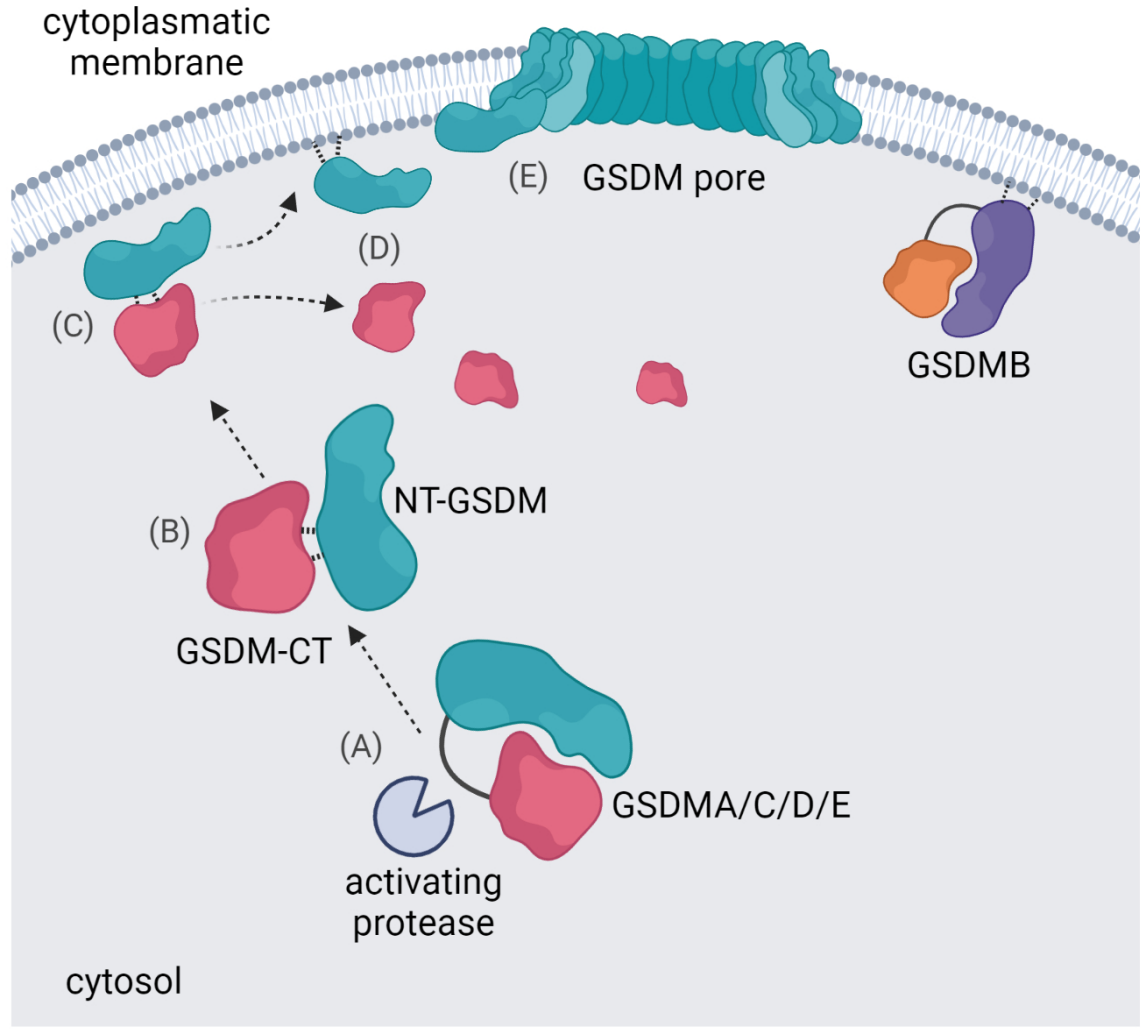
Gasdermin pore formation. An activating protease cleaves the gasdermin protein in the linker region between NT-GSDM and GSDM-CT (A) the NT-GSDM and GSDM-CT subunits remain associated in a complex (B) the NT-GSDM/GSDM-CT complex interacts with a membrane (C) and dissociates upon this interaction (D) NT-GSDM subunits oligomerize and form pores (E) GSDMB has a unique ability to interact with the cytoplasmatic membrane in its full-length form.
